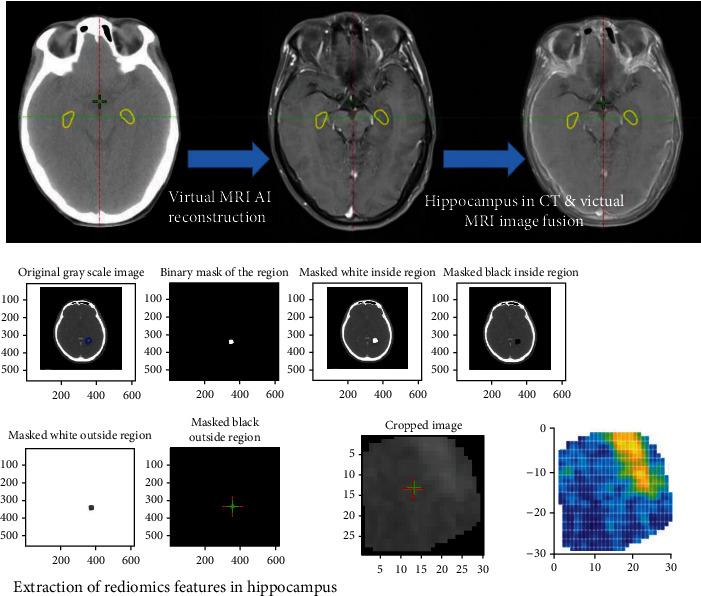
The process of automatically delineating the hippocampus ROI from CT and radiomics feature extraction.
